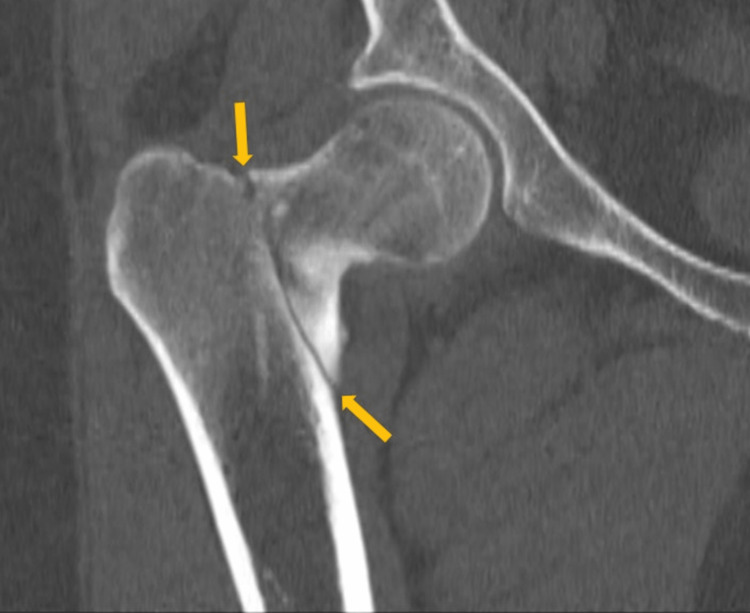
A CT scan showing the right intertrochanteric fracture of the right femur (yellow arrows)
